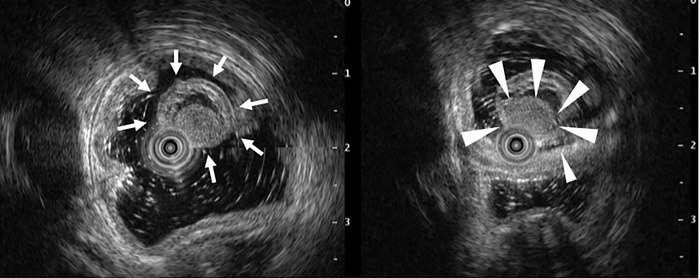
Endoscopic ultrasonography of the duodenal duplication cyst. A 10‐mm cystic tumor (white arrow) of low echogenicity that included an isoechoic circular structure (white arrowhead). The first layer of the cyst lumen was hyperechoic, the second layer was hypoechoic, the third layer was hyperechoic, the fourth layer was hypoechoic, and the fifth layer was hyperechoic. The circular structure was thought to be nodules and debris
